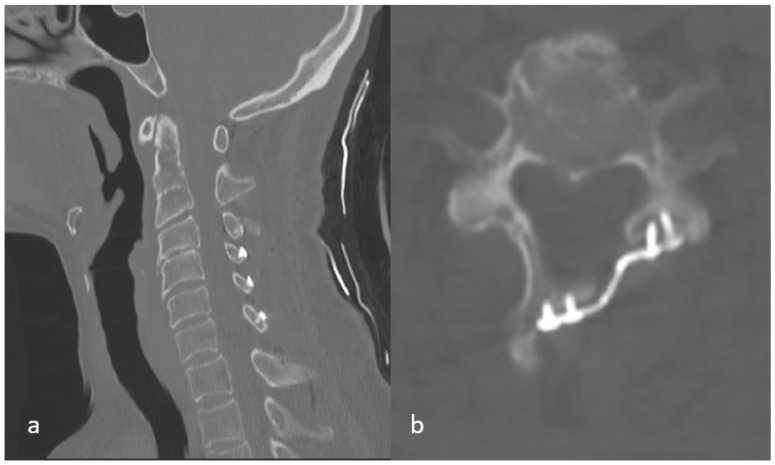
Postoperative CT scan: (a) in the sagittal image, the canal has been widened with the help of mini plates; (b) in the axial image, the lamina has been lifted with the help of screws fixed to the lateral mass and lamina.
